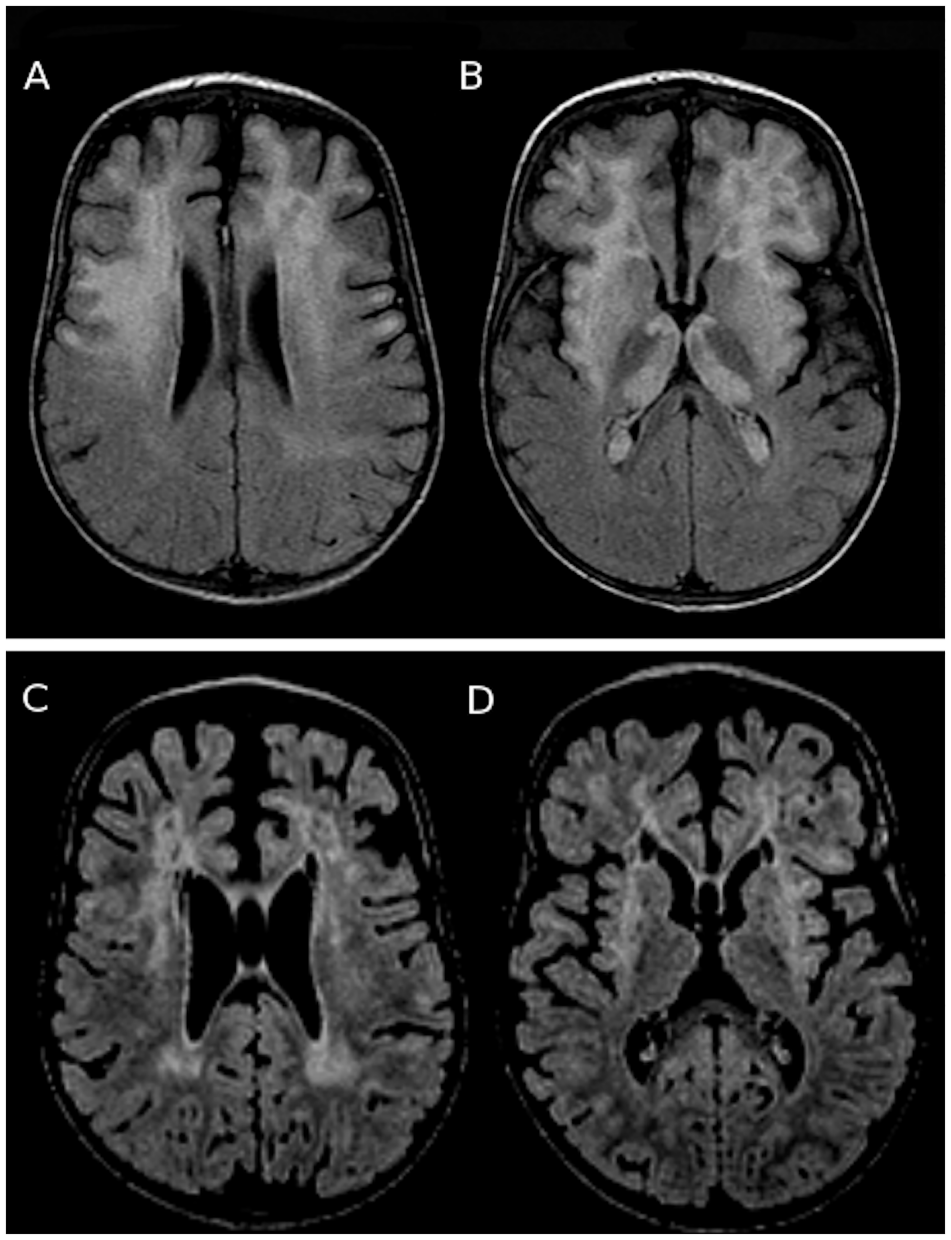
Brain MRIs of the son before and after treatment of the meningoencephalitic stage of trypanosomiasis. (A, B) Initial brain MRI in June 2013 (axial fluid attenuated inversion recovery [FLAIR]-weighted images) showing extensive and confluent hyperintensities in the frontotemporal white matter and basal ganglia (especially in the thalami and lenticular nuclei), with global brain atrophy for a 13-month-old patient. (C, D) Brain MRI in January 2014, 6 months after treatment (3D-T1 and FLAIR-weighted images), showing resolution of the thalamic lesions, diminution of the lesions in the lenticular nuclei and white matter, and increased secondary atrophy.
